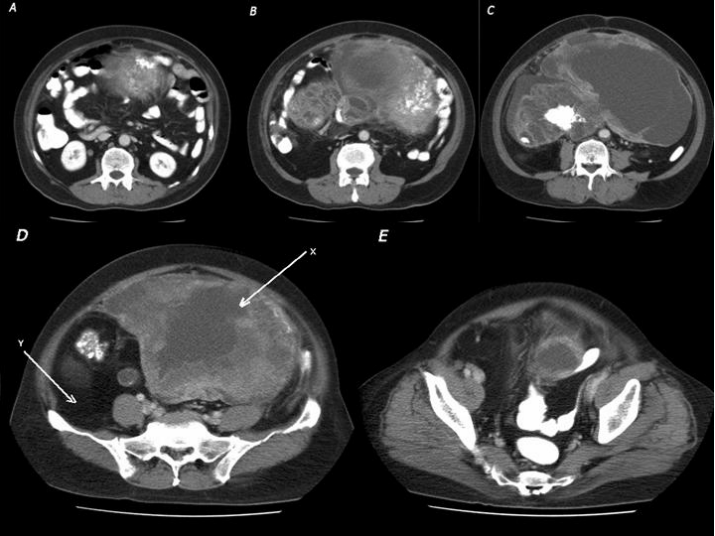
CT appearance at multiple cuts (A – E) of a huge retroperitoneal mass with lipomatous and nonlipomatous components. The nonlipomatous component (arrow X, Panel D) contains calcific elements. Note the posterior extension of the lipomatous component (arrow Y, Panel D), which extends superiorly (Panels A – C). The exact boundaries of this component are difficult to appreciate on CT.
